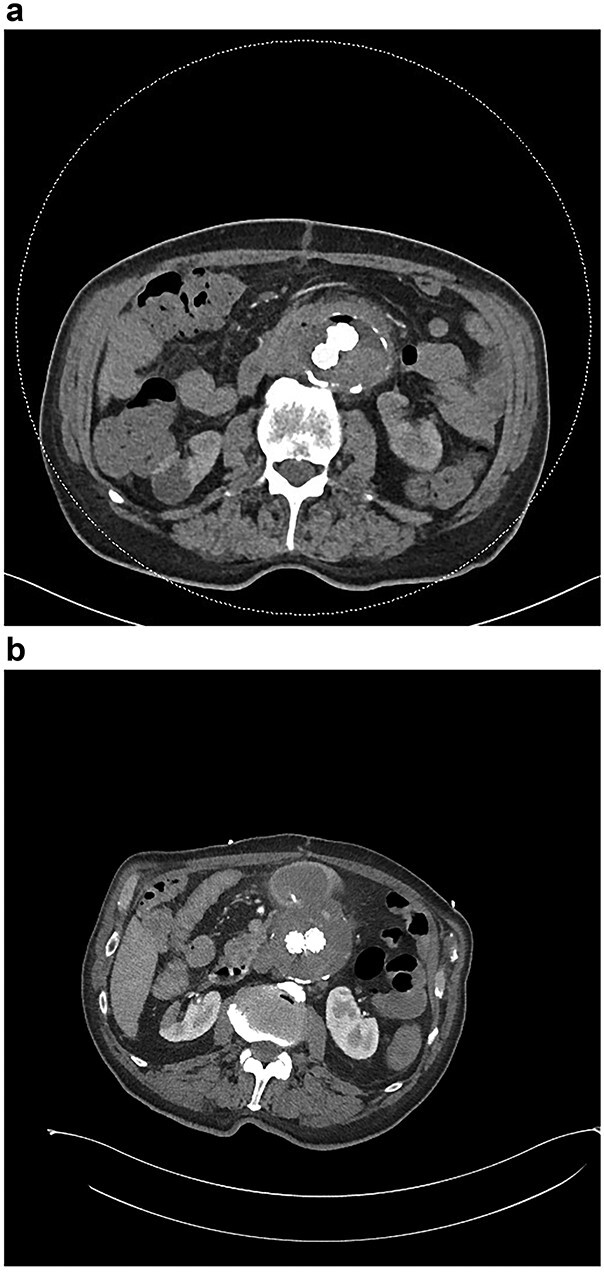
(a) CT angiogram demonstrating new gas in the aneurysmal sac 6 weeks post-repair; (b) CT angiogram just prior to the second repair, demonstrating ongoing endoleak and continuity of the duodenum and sac; part of the duodenal clip is visible.
